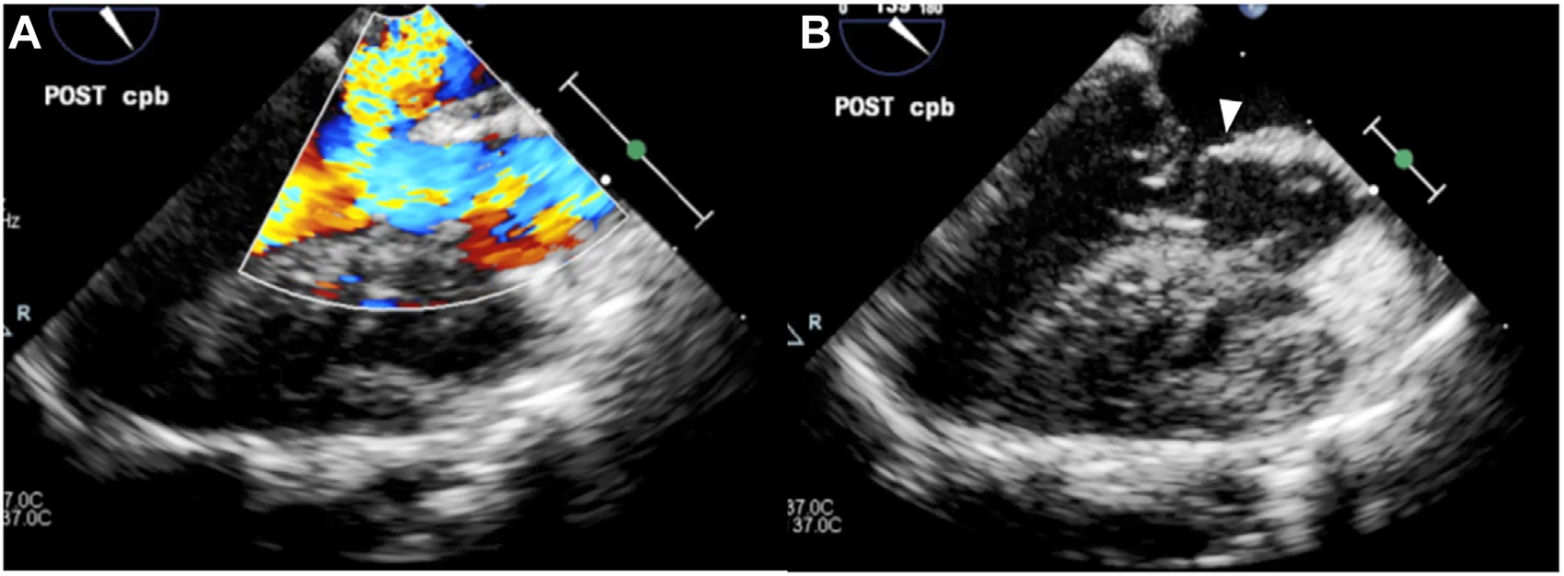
Intraoperative transesophageal echocardiography showed (A) severe mitral regurgitation and (B) systolic anterior motion of anterior leaflet of mitral valve after weaning from cardiopulmonary bypass (cpb; arrowhead).
